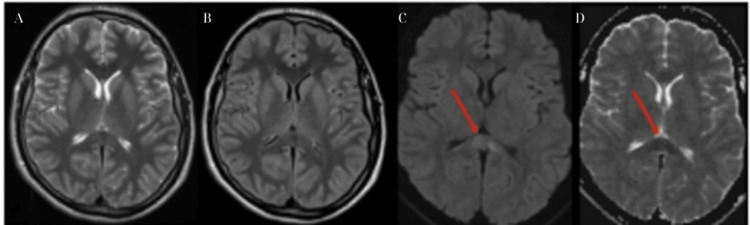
Initial MRI imaging: (A) initial T2 sequence appeared normal; (B) meningeal and linear-perivascular enhancements were observed in the T1 sequences following gadolinium administration; (C) signal increase in DWI; (D) decrease in the ADC map indicated restricted water in the splenium of the corpus callosum, indicative of cytotoxic edema (red arrows). DWI: diffusion-weighted imaging; ADC: apparent diffusion coefficient
